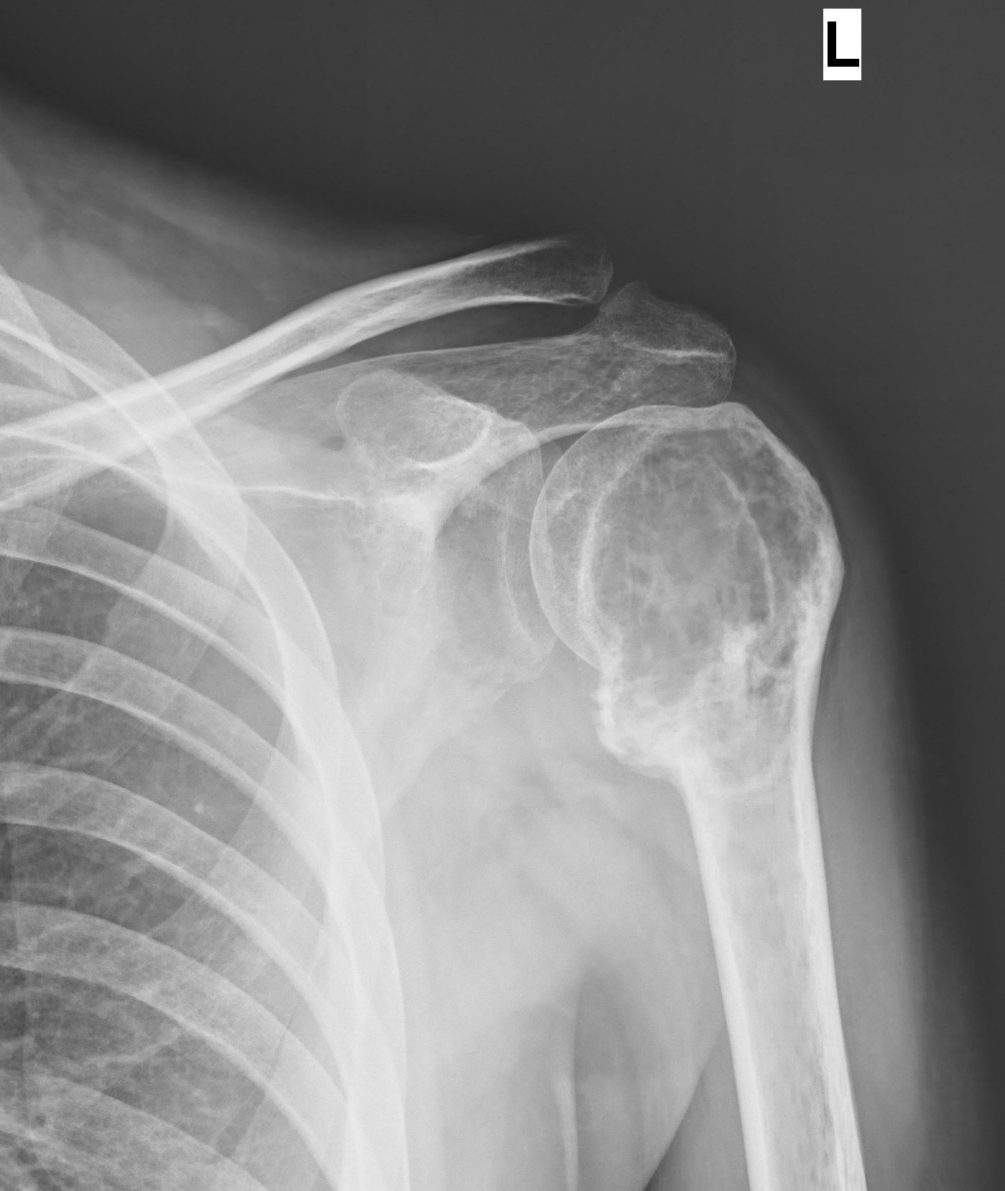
Post-denosumab therapy radiograph of the left shoulder (Case #1) showing patchy ossification and sclerotic rimming of the lesion in the proximal humerus.
